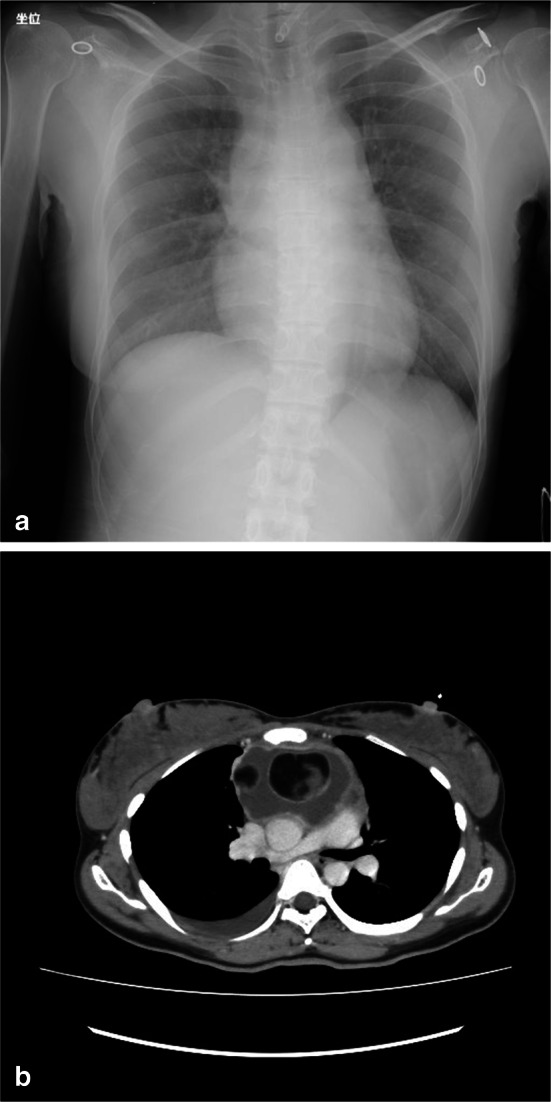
a Chest X-ray taken on admission displaying bilateral mediastinal widening. b Chest CT displaying a fat-containing cystic mass with solid components in the anterior mediastinum, and pleural effusion in the right side
